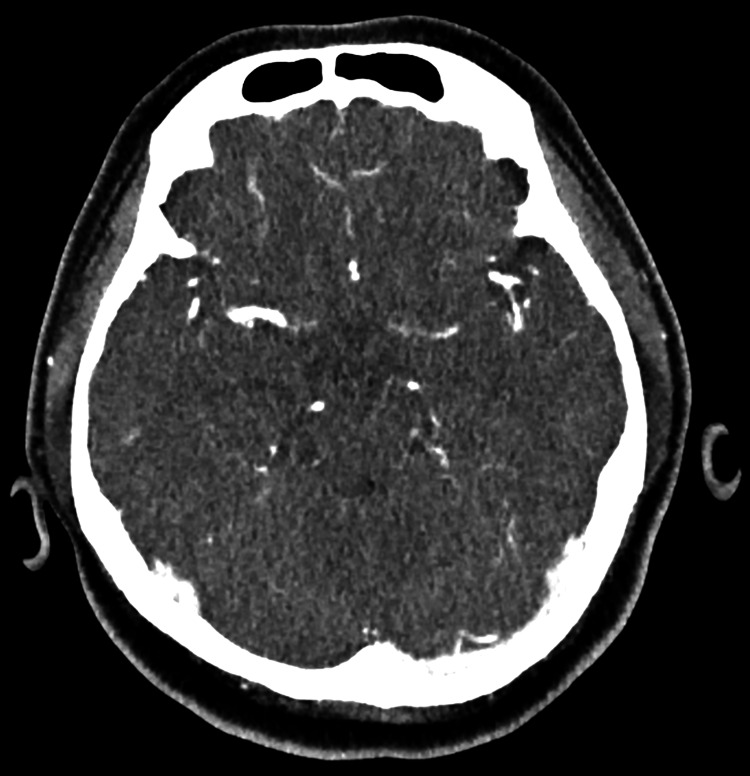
CTA of the brain showing normal arterial opacification with no evidence of large-vessel occlusion
